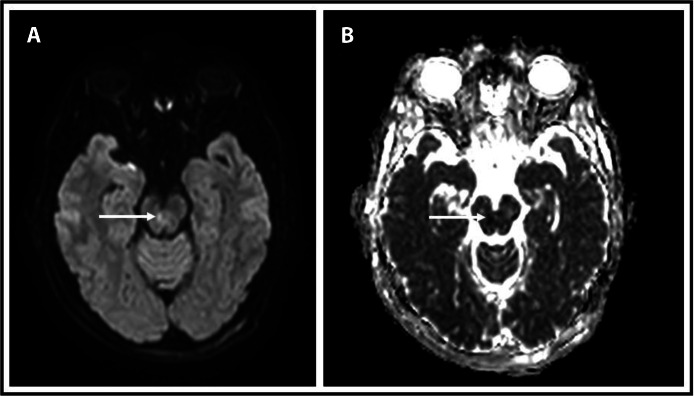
Brain MRI demonstrating an acute right midbrain ischemic infarction. (A) Diffusion-weighted imaging (DWI) demonstrates focal hyperintensity within the right midbrain (arrow), indicating restricted diffusion. (B) Corresponding ADC map shows signal hypointensity at the same location (arrow), confirming true diffusion restriction and supporting the diagnosis of acute ischemic stroke. The DWI–ADC mismatch is characteristic of recent infarction and allows early detection of posterior circulation ischemic lesions that may be occult on conventional imaging.
